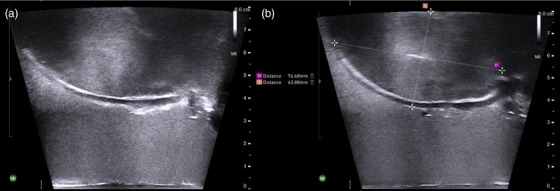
(a) Sagittal TRUS of the uterus with (b) displaying the measurements (in mm) of the uterus, as measured on the BK Specto US system. TRUS, transrectal ultrasound.
